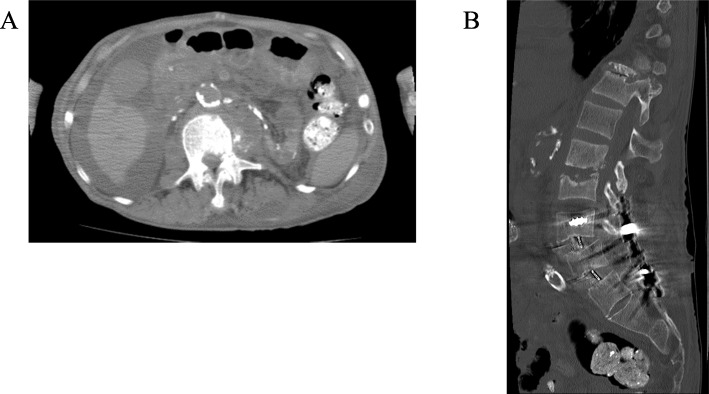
Computed tomography of lumbar spine and psoas abscess on day 5, the day after meropenem and vancomycin were initiated. a A coronal image showing low density area in the lateral part of left psoas major; b a sagittal image showing several bone pieces in the intervertebral area of L2/L3 and swelling of the disc. Although he had received two prior surgeries in his lower lumbosacral spine from L4 to S2, there was neither fracture nor abscess around the instruments
